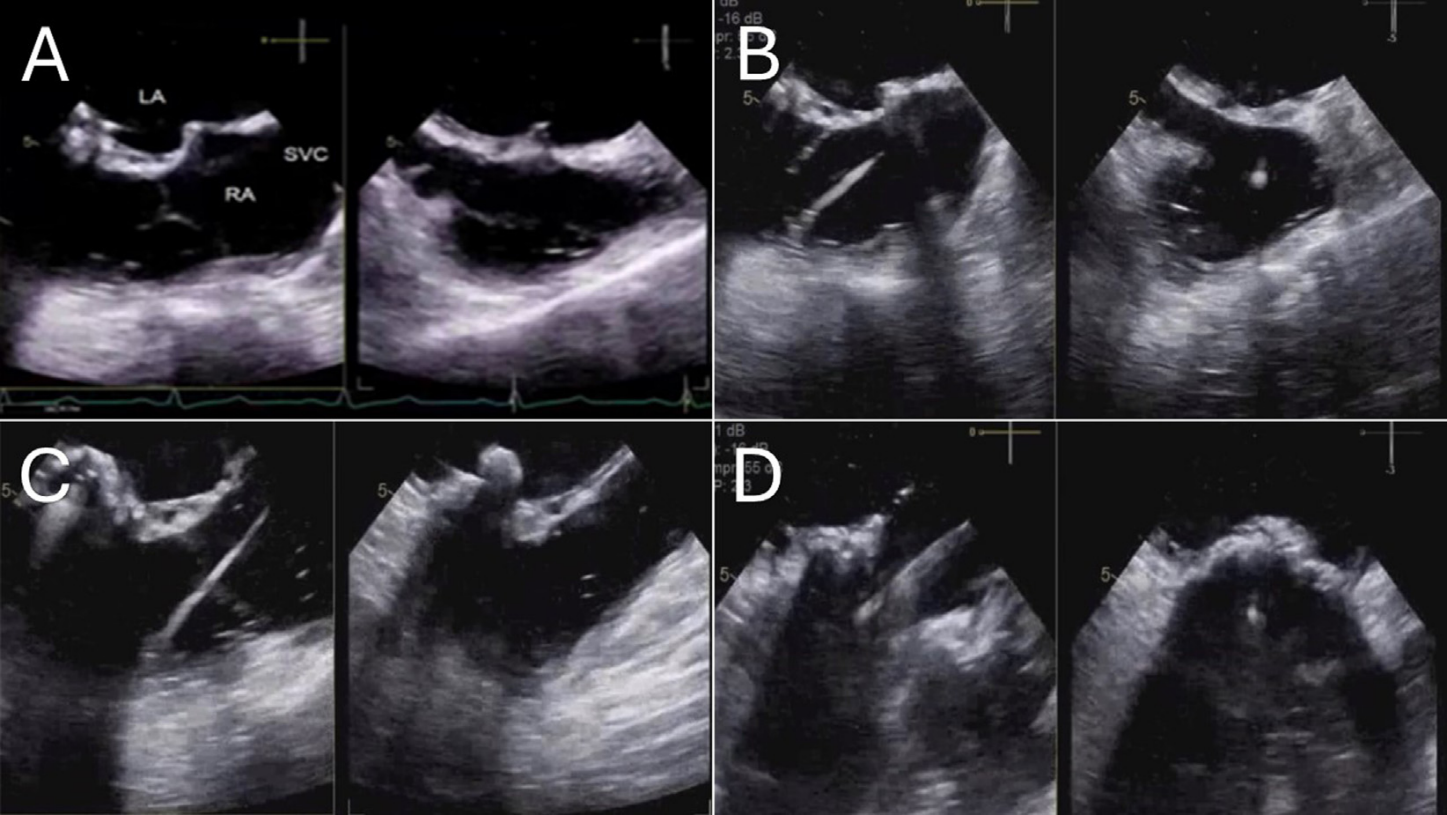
Transesophageal Echocardiography: Multiple Views These views, captured at each step of the procedure, outline the process of placing the new valve. (A) Transseptal puncture. (B) Advancement of the pacing wire. (C) Rail placement for valve deployment. (D) Positioning and ballooning of the stented valve. SVC = superior vena cava; other abbreviations as in Figures 1 and 2.
